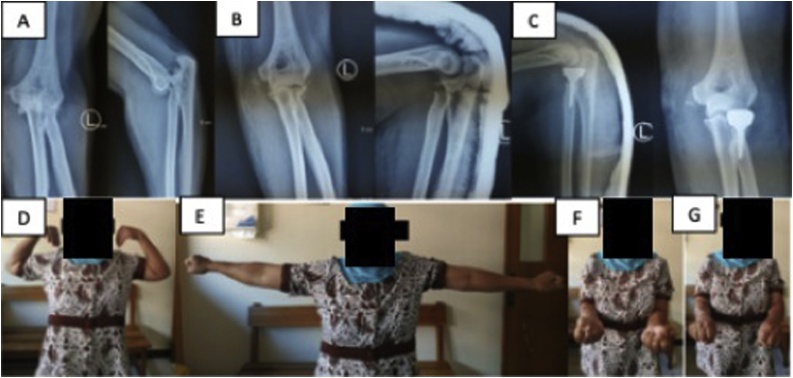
A Pre-operation AP/Lateral X-ray of the left elbow from the second patient before closed reduction was performed. The elbow was dislocated and the radial head was fractured. Thus, it is Mason type IV injury. Fig. 2B AP/Lateral X-ray of the left elbow from the same patient after closed reduction and application of posterior slab. Fig. 2C Post-operation AP/Lateral X-ray of the left elbow from the same patient. This patient was managed with prosthetic replacement of the radial head. Fig. 2D–G Active ROM evaluation of the same patient; elbow flexion of 140°; elbow extension of 5°; elbow supination of 80°; elbow pronation of 70°.
